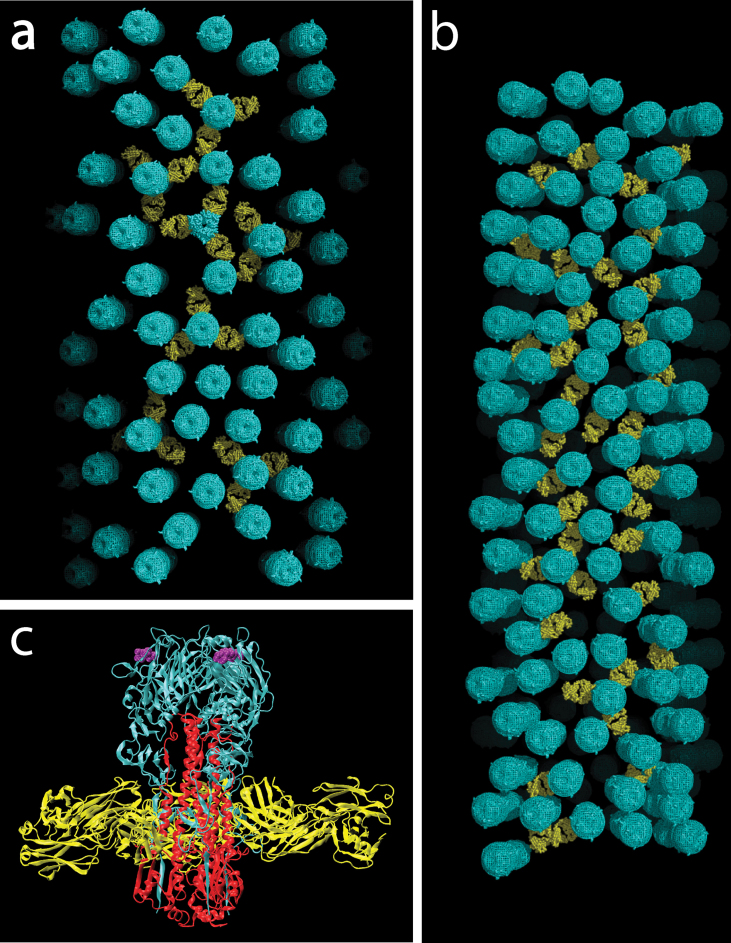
(a) Glycoprotein positions for an X-31 virion with 13% fractional surface volume. Glycoproteins are represented as cylindrically averaged molecules (cyan) to reflect orientational uncertainty. Several models show 3 FI6 Fabs (yellow) bound to the HA stem. (b) Glycoprotein positions for a Udorn virion with 15% fractional volume. (c) X-ray model of HA trimer with FI6 Fabs bound to stem domain [7].
